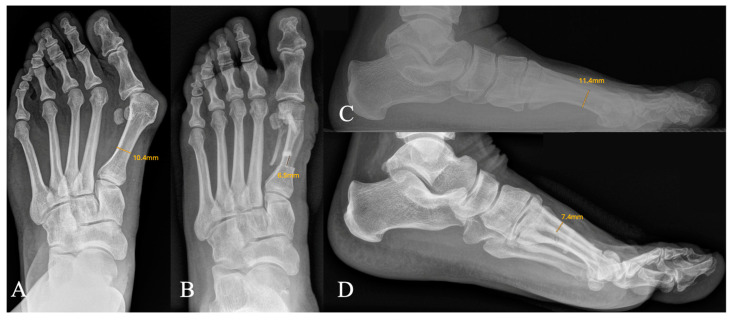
Fracture analysis of patients treated with allograft bone screws in scarf surgery. (A) Preoperative dorsoplantar X-ray showing the minimal diaphyseal width (B) postoperative dorsoplantar X-ray showing distance from the proximal screw to the proximal osteotomy (C) preoperative lateral X-ray showing the minimal diaphyseal metatarsal depth (D) postoperative lateral X-ray showing the distance between the proximal plantar osteotomy and the dorsal diaphyseal metatarsal cortical bone.
